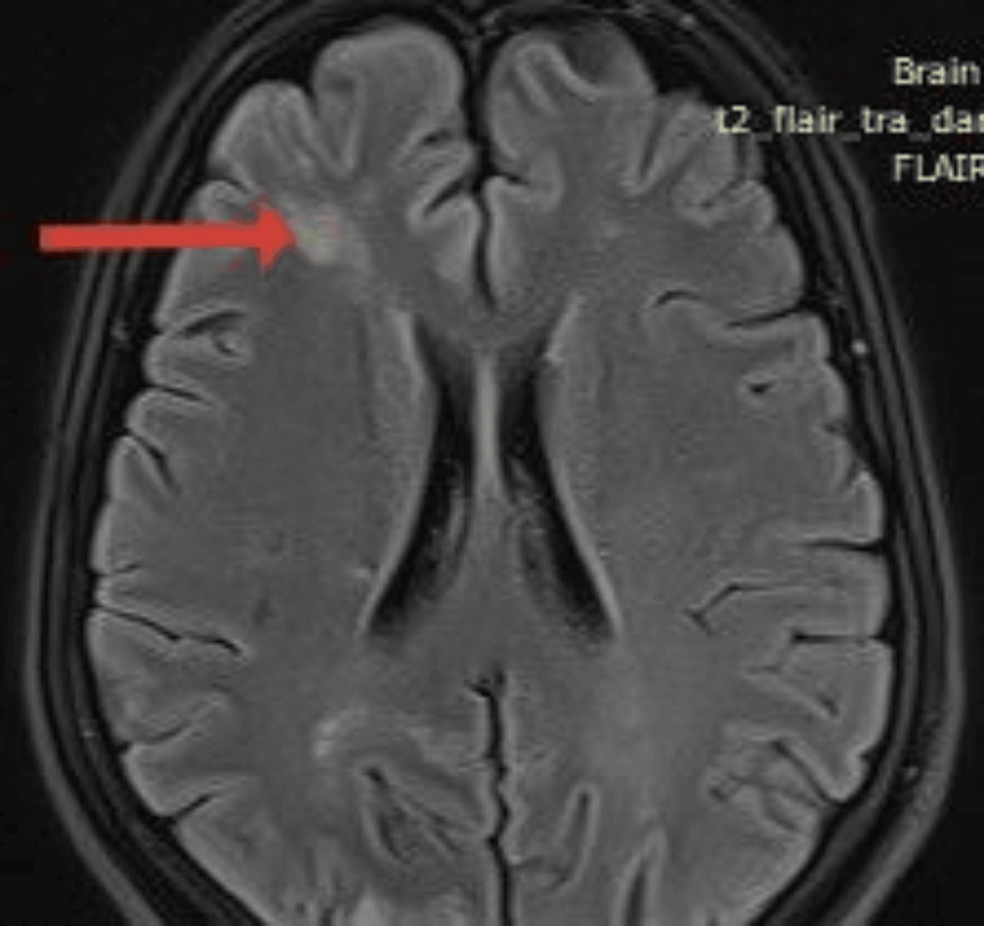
MRI of the brain showing bilateral acute to subacute infarction involving the frontal-parietal, occipital, and left cerebellum
